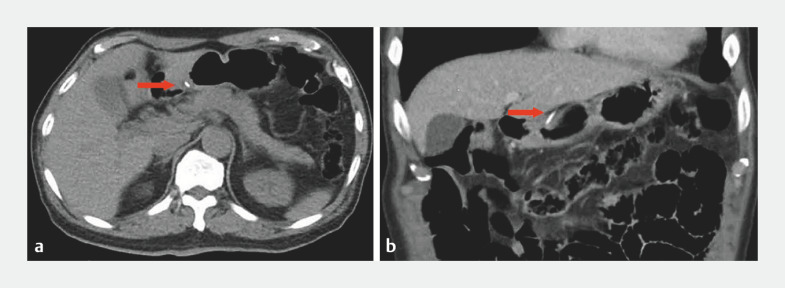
Computed tomography images showing the location and depth of the fishbone (red arrow) on:
